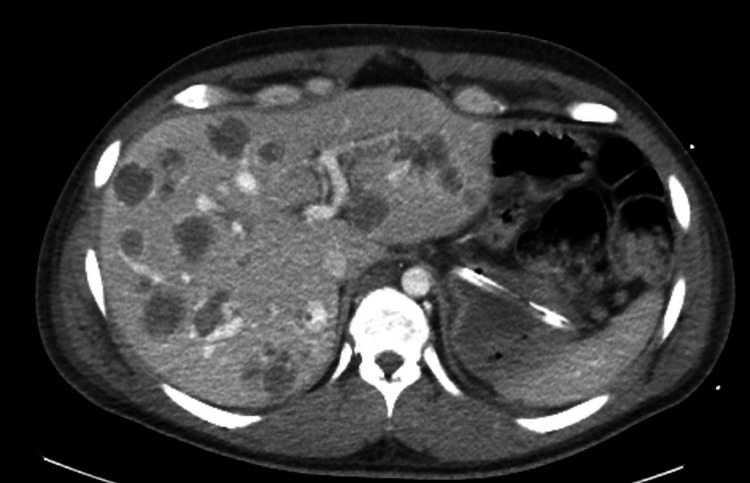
CT of the abdomen showing a significant increase in the size of all hepatic metastatic lesions, with lesions appearing more hypodense, representing hemorrhage
